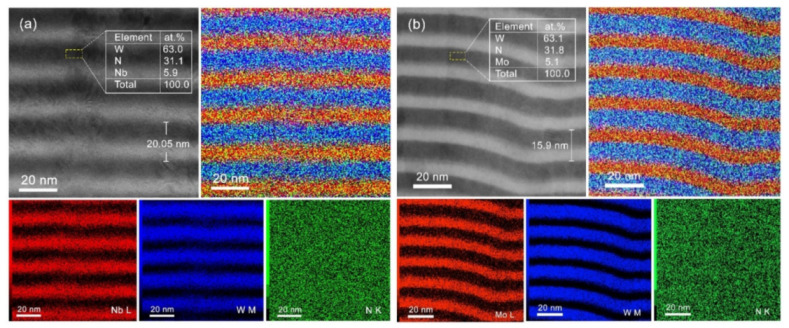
Cross-sectional BF STEM images of (a) WN/NbN and (b) WN/MoN multilayer coatings with element concentrations in WN layers. STEM-EDX maps of Nb L/Mo L, W M, and N K with the superposition X-ray maps.
